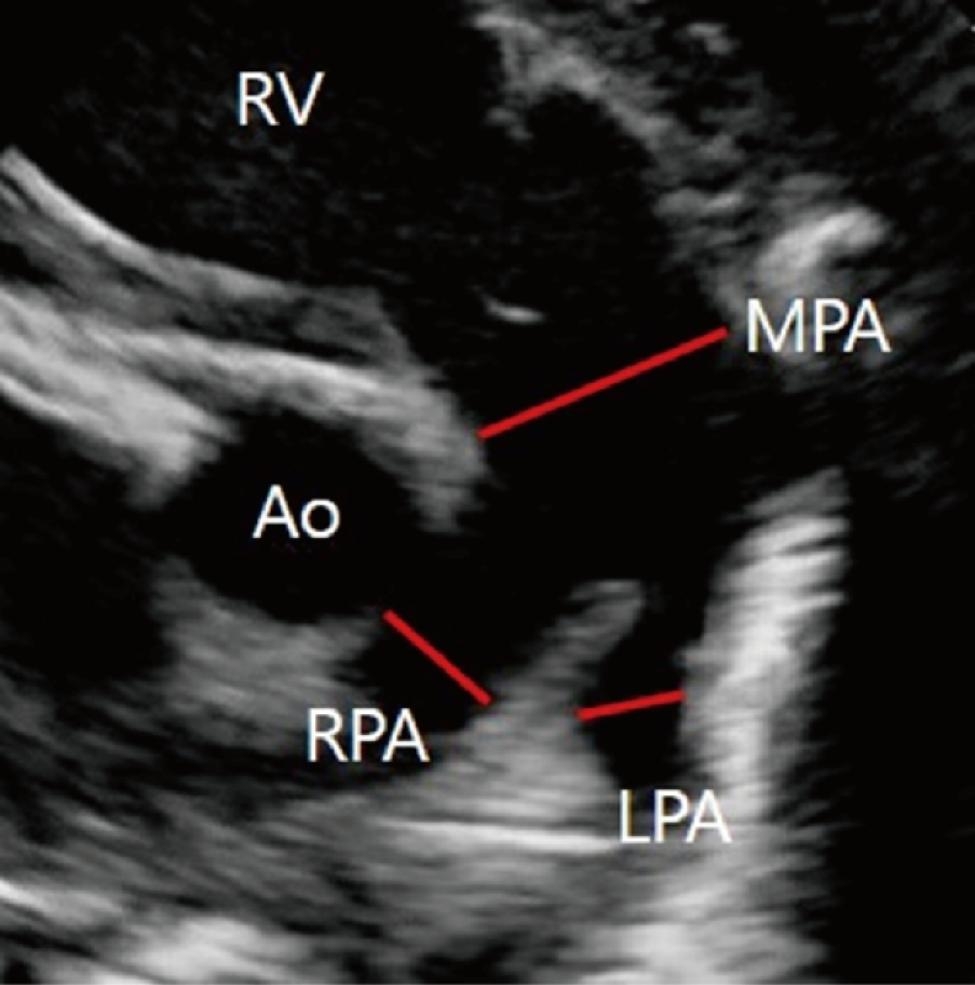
Measuring the diameters of the main pulmonary artery (MPA) and the proximal branch pulmonary artery in a parasternal short-axis view Abbreviations: Ao, Aorta; MPA, main pulmonary artery; RPA, right pulmonary artery; LPA, left pulmonary artery; RV, right ventricle
